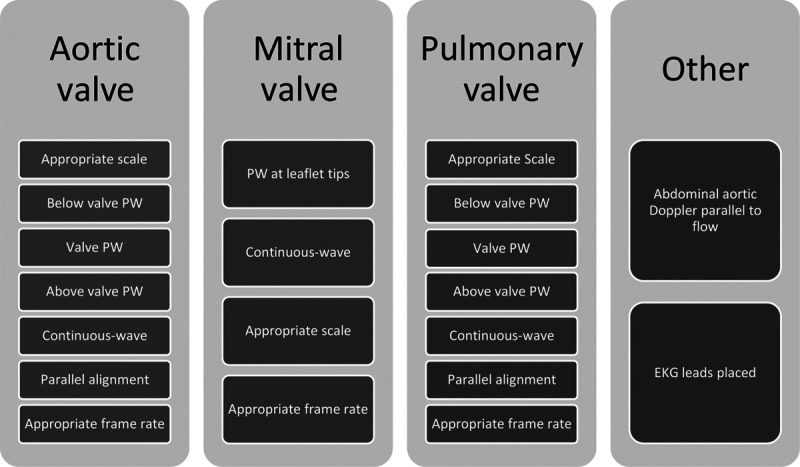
The Doppler scoring system. Each component received one point for a total of 20 points. PW, pulsed-wave Doppler.
